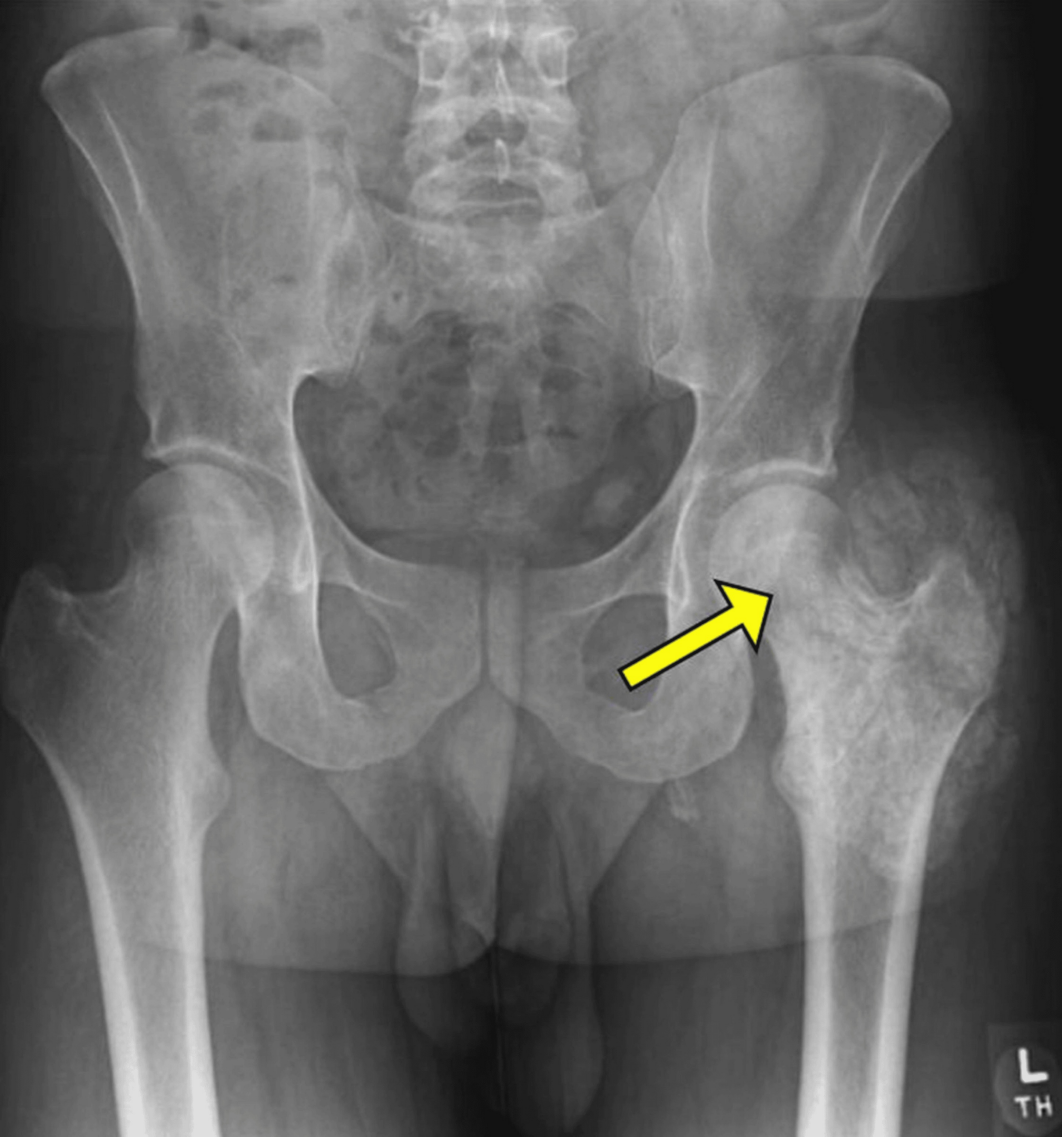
X-ray pelvis: amorphous calcific densities are shown by the yellow arrow
